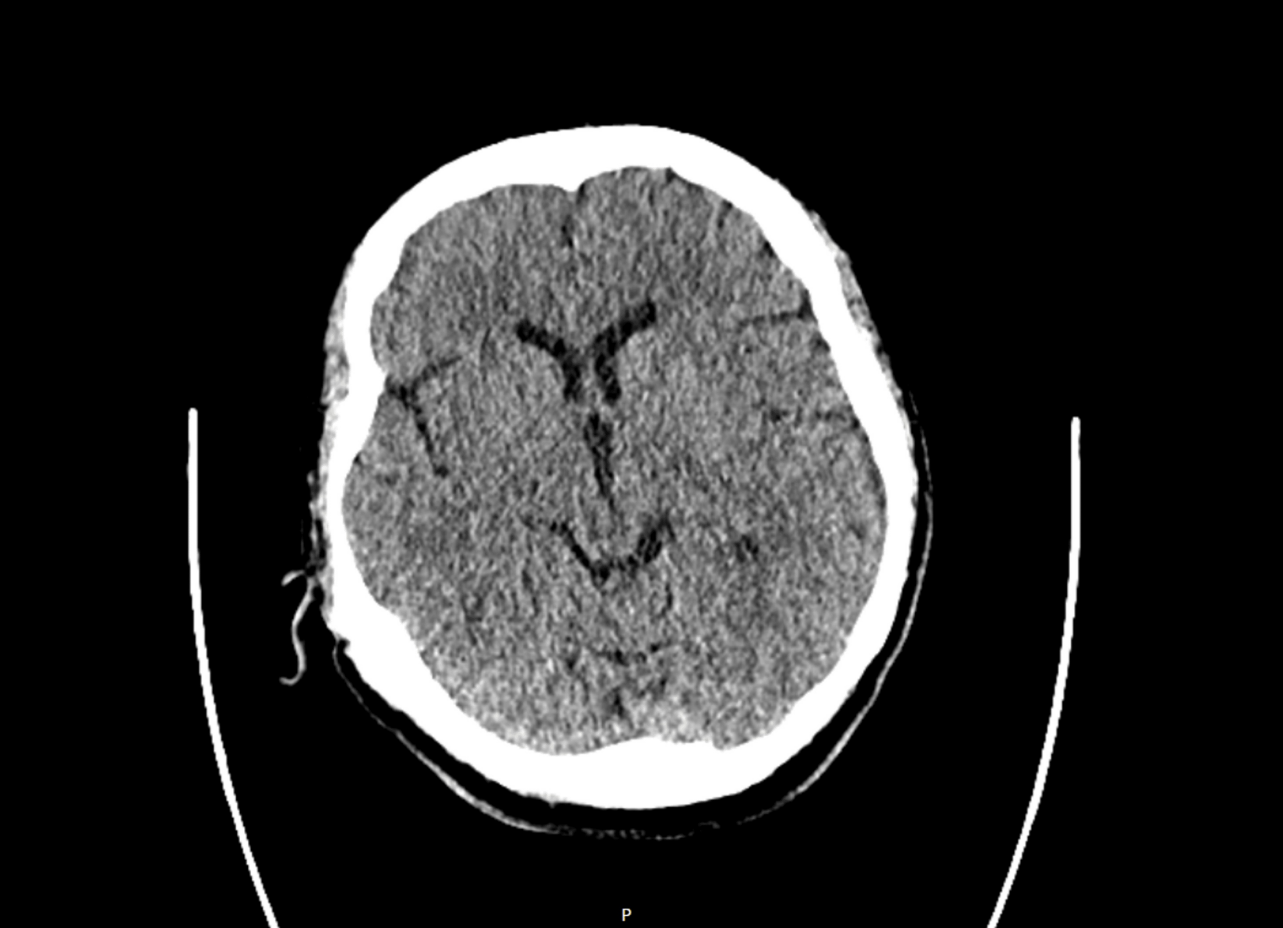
Repeat CT head demonstrating no intracranial abnormalities
